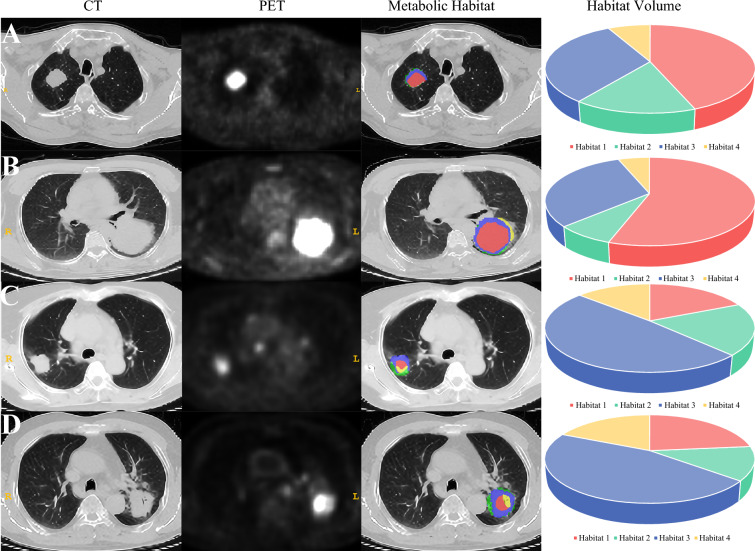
Representative ^18^F-FDG PET/CT and metabolic habitat images of two PD-L1-positive expression patients (a, b) and two PD-L1-negative expression patients (c, d). Note: (1) We mapped each metabolic habitat onto CT images, annotating each habitat subregion. (2) Habitat 1 (red) represented high-glycolytic/high-density habitats; Habitat 2 (green) represented low-glycolytic/low-density habitats; Habitat 3 (blue) represented low-glycolytic/high-density habitats; and Habitat 4 (yellow) represented high-glycolytic/low-density habitats. ^*18*^*F-FDG PET/CT*
